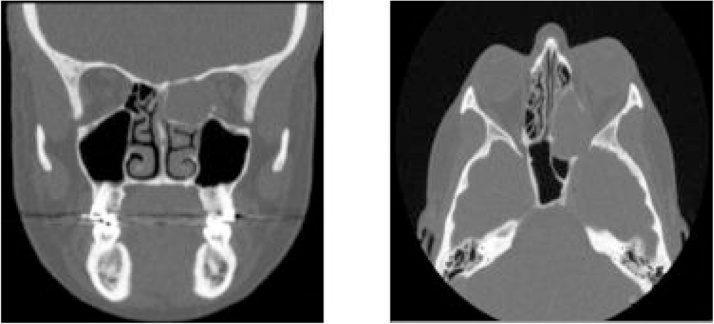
CT Sinuses, coronal view (a) and axial view (b) demonstrating a large, left-sided ethmoid mucocoele extending through the left optic canal and compromising the left optic nerve. CT sinuses: Coronal view (figure a, left) and Axial view (figure b, right) demonstrating a large, left-sided Ethmoid mucocoele extending through the left Optic canal and compressing the left Optic nerve. 127 × 57 mm (150 × 150 DPI).
